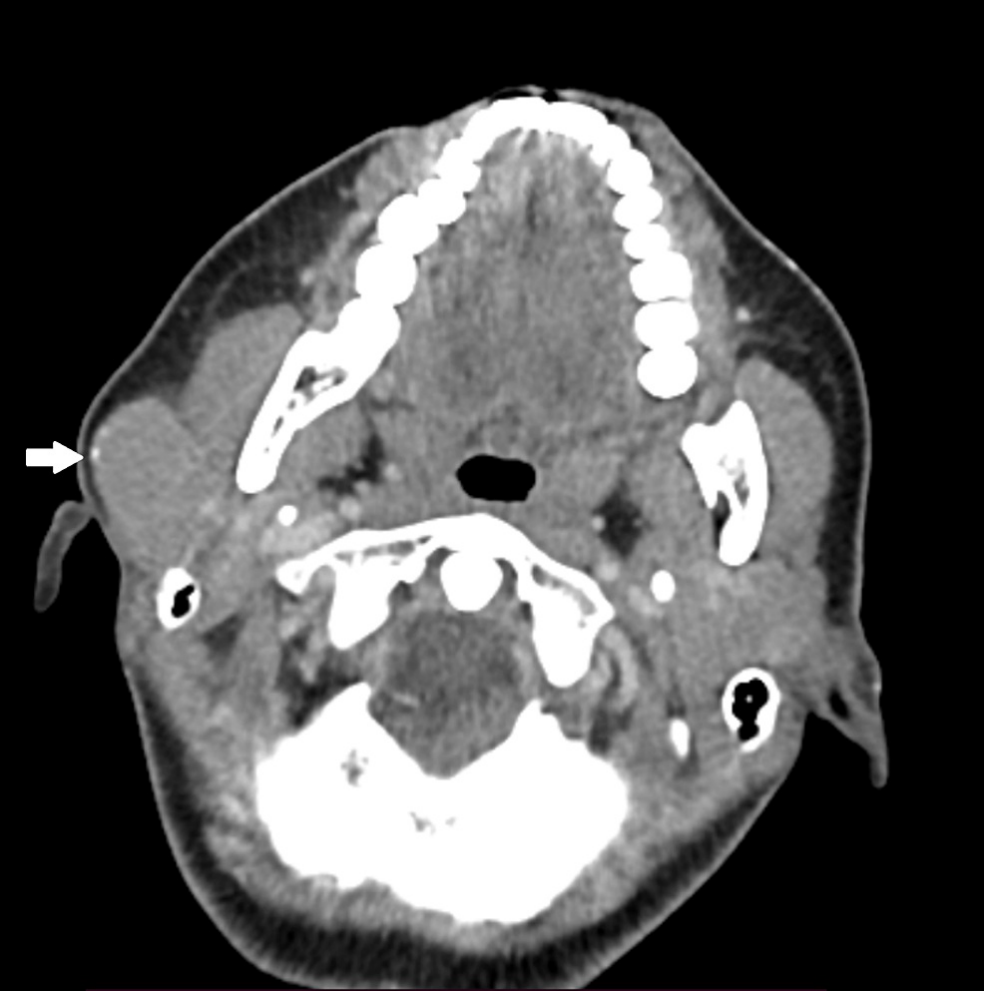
Axial CT image of the parotid gland An axial CT image of the parotid gland demonstrates a homogeneously enhancing lesion at the superficial lobe of the right parotid gland.
